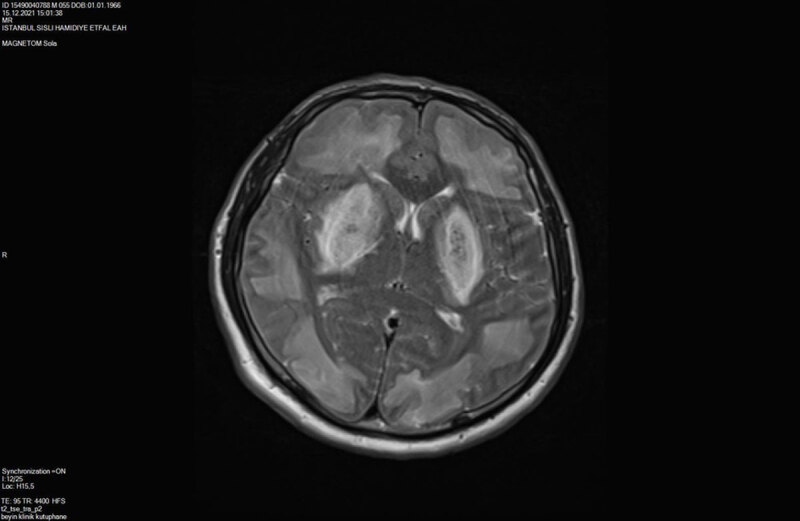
FSE T2-weighted image, bilateral symmetric extensive signal intensity increase along both cerebral hemispheres subcortical region and also bilateral putamina.
